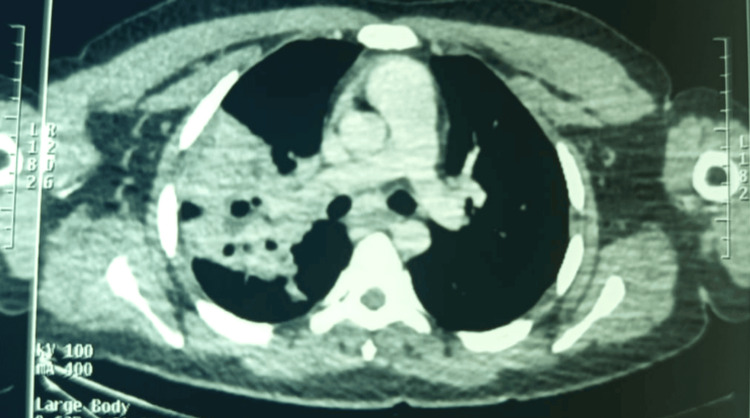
Repeat computed tomography imaging showing enlargement of the cavitary lesion
